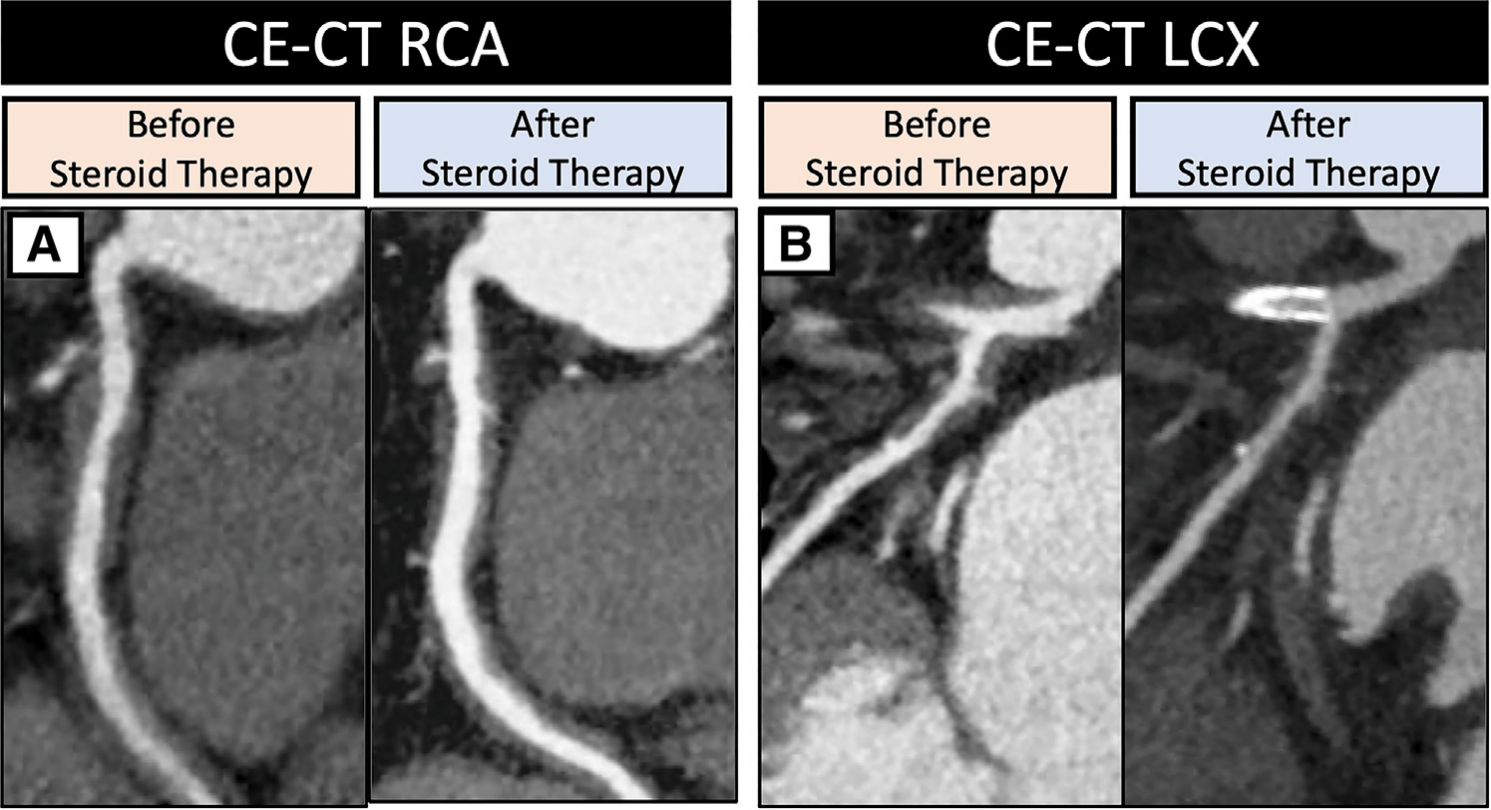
Contrast-Enhanced Computed Tomography on the Third Month of Corticosterone Therapy The wall thickening was significantly decreased in (A) the right coronary artery and (B) the left circumflex artery. CE-CT = contrast-enhanced computed tomography; LCX = left circumflex artery; RCA = right coronary artery.
